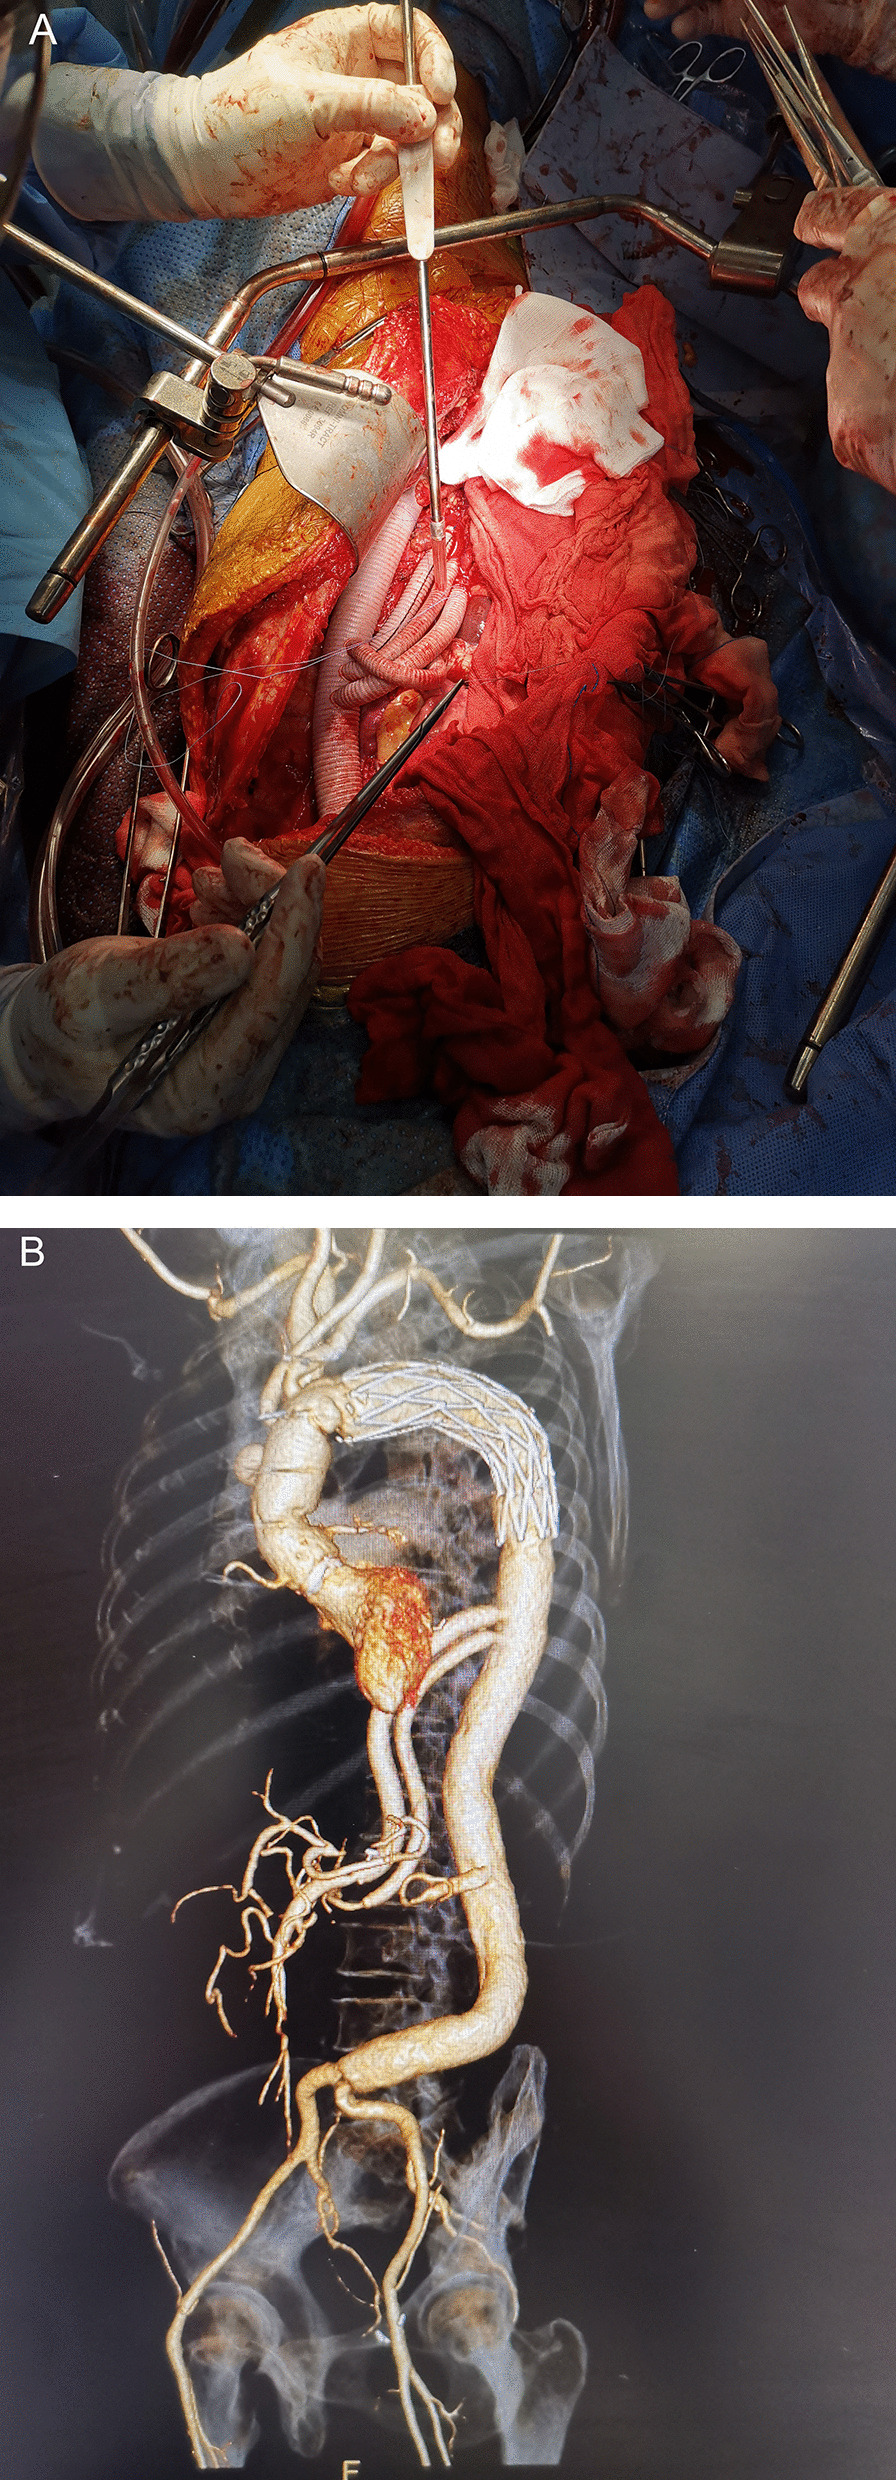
This patient successfully received the second-stage of thoracic–abdominal aortic replacement surgery (A). The postoperative CT (B) showed that the artificial blood vessel was normal in shape, the whole thoracic–abdominal aortic aneurysm was completely removed, and the revascularization of important organs was satisfactory
